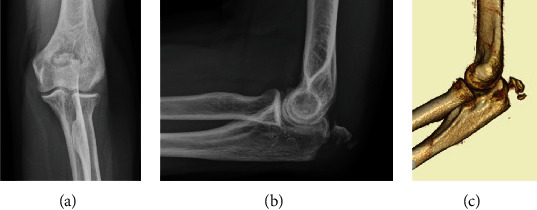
Plain radiography and computed tomography (CT) images of the left elbow. Plain radiography revealed an avulsion fracture of the olecranon process (a, b). CT shows small, avulsed fragments with comminuted bone (c).
